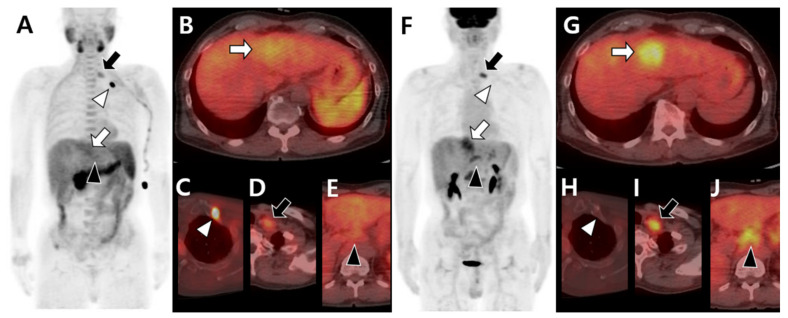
Different tracer avidity according to the metastatic organ in the same patient with hepatocellular carcinoma before therapy. ^11^C-acetate positron emission tomography (PET)/computed tomography (CT) (A–E) shows mild uptake in the primary hepatic tumor (white arrow in A,B). The metastatic bone lesion in the left first rib shows intense acetate uptake (white arrowhead in A,C) but less acetate uptake in metastatic lymph nodes of the left supraclavicular (black arrow in A,D) and common hepatic (black arrowhead in A,E) areas. ^18^F-fluorodeoxyglucose (^18^F-FDG) PET/CT (F–J) shows intense uptake in primary hepatic tumors (white arrow in F,G). There is no significant FDG uptake in the left first rib (white arrowhead in F,H) but intense uptake in the left supraclavicular (black arrow in F,I) and common hepatic (black arrowhead in F,J) lymph nodes.
